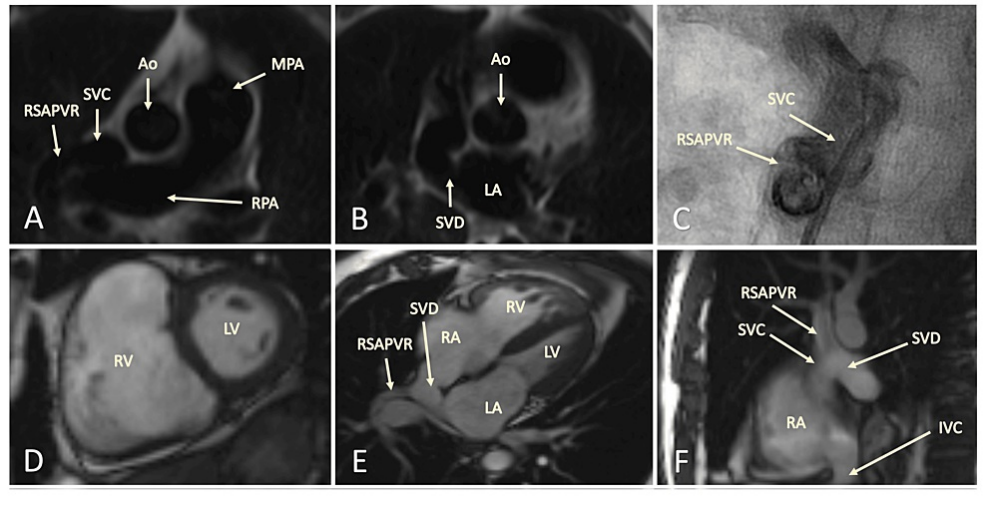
CMR images confirming diagnosis of SVD with RSAPVR (A-B) CMR T1 weighted images revealing the RSAPV joining the SVC and the SVASD. (C) Selective angiography of the RSAPV, SVC, and SVASD junction. (D) CMR T2 weighted images demonstrating severe dilation of the RV on diastole. (E-F) 4 chamber view depicting the junction of the RSAPVR, SVC, and SVD. CMR: cardiac magnetic resonance imaging; RV: right ventricle; RA: right atrium; LV: left ventricle; LA: left atrium; SVD: sinus venosus defect; SVC: superior vena cava; RSAPVR: right superior anomalous pulmonary venous return.
